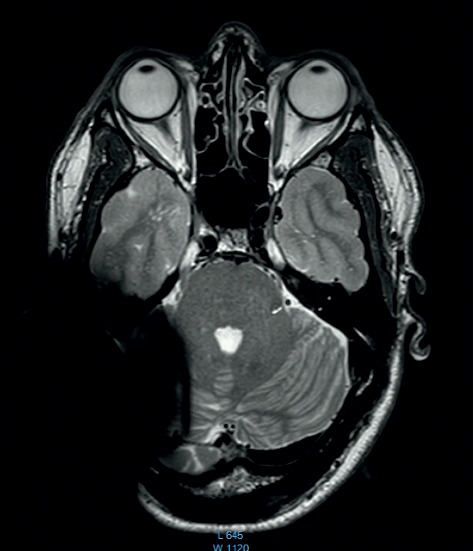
MRI pattern of the axial plain of a 3 Tscanning Advanced Bionics 3D Implant.
